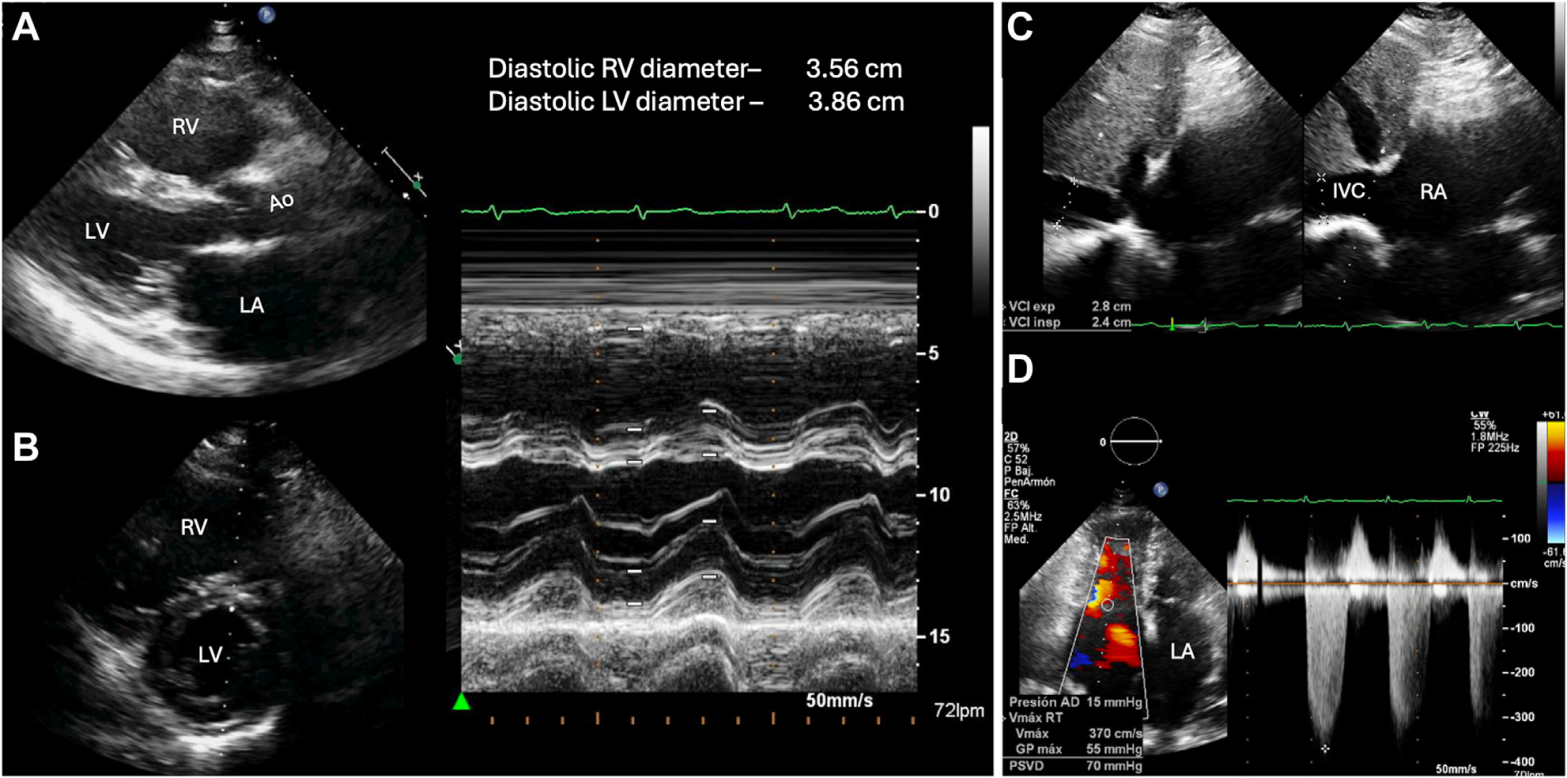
Two-dimensional TTE-guided M-mode, parasternal long-axis (A) and short-axis (B) views, demonstrate RV dilation and paradoxical movement of the IVS. Subcostal view of the inferior vena cava (C) during inspiration (*left*) demonstrates less than 50% inspiratory collapse. Right ventricle–focused apical 4-chamber view (D) with color-flow Doppler-guided continuous-wave Doppler, reveals a peak TR velocity of 3.7 m/sec and estimated RV systolic pressure of 70 mm Hg. *Ao*, Aorta; *IVC*, inferior vena cava; *LA*, left atrium; *LV*, left ventricle; *RA*, right atrium; *RV*, right ventricle.
